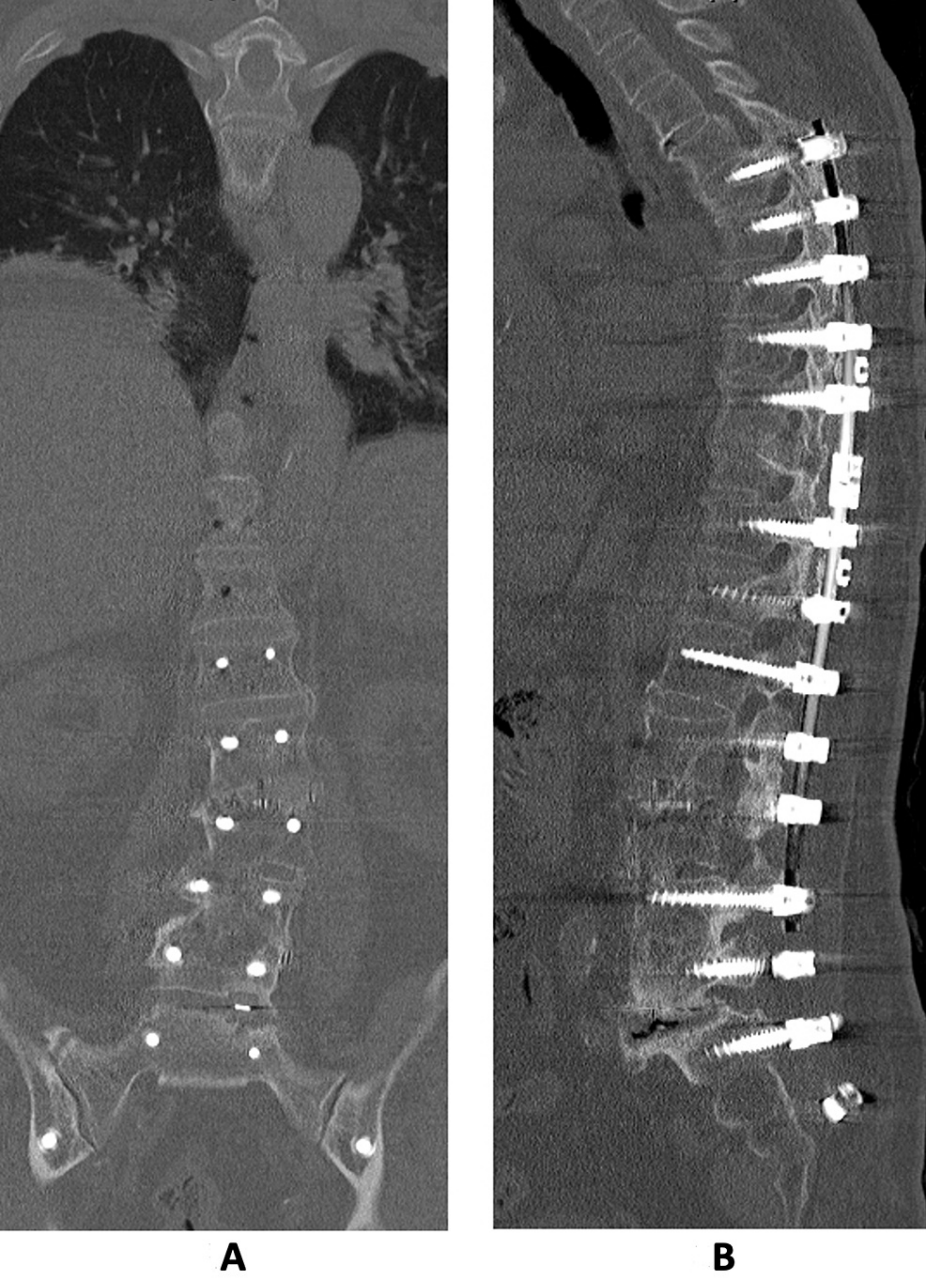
A: Coronal CT images demonstrating pseudoarthrosis at L5/S1 and absence of the broken rod on the left. B: Sagittal CT image showing pseudoarthrosis at L5/S1.
